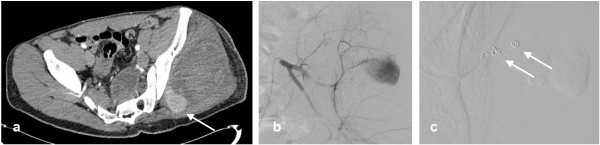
a) Axial arterial phase contrast enhanced CT in a 23 year old man following a stab wound to the left buttock demonstrates haematoma within the gluteus muscles. Contrast enhancement medially (arrow) represents active haemorrhage from the superior gluteal artery (Somatom sensation, 24 slice,Siemens, Erlangen, Germany). b) A Cobra catheter was negotiated into the posterior (somatic) left internal iliac artery from an ipsilateral approach. Active haemorrhage from a branch of the superior gluteal artery was demonstrated. c) A microcatheter system (Progreat) was negotiated into the bleeding vessel and 2 microcoils (Boston Scientific vortex fibred) were deployed (arrows). This completely abolished the bleeding with good perfusion of the buttock post procedure.
